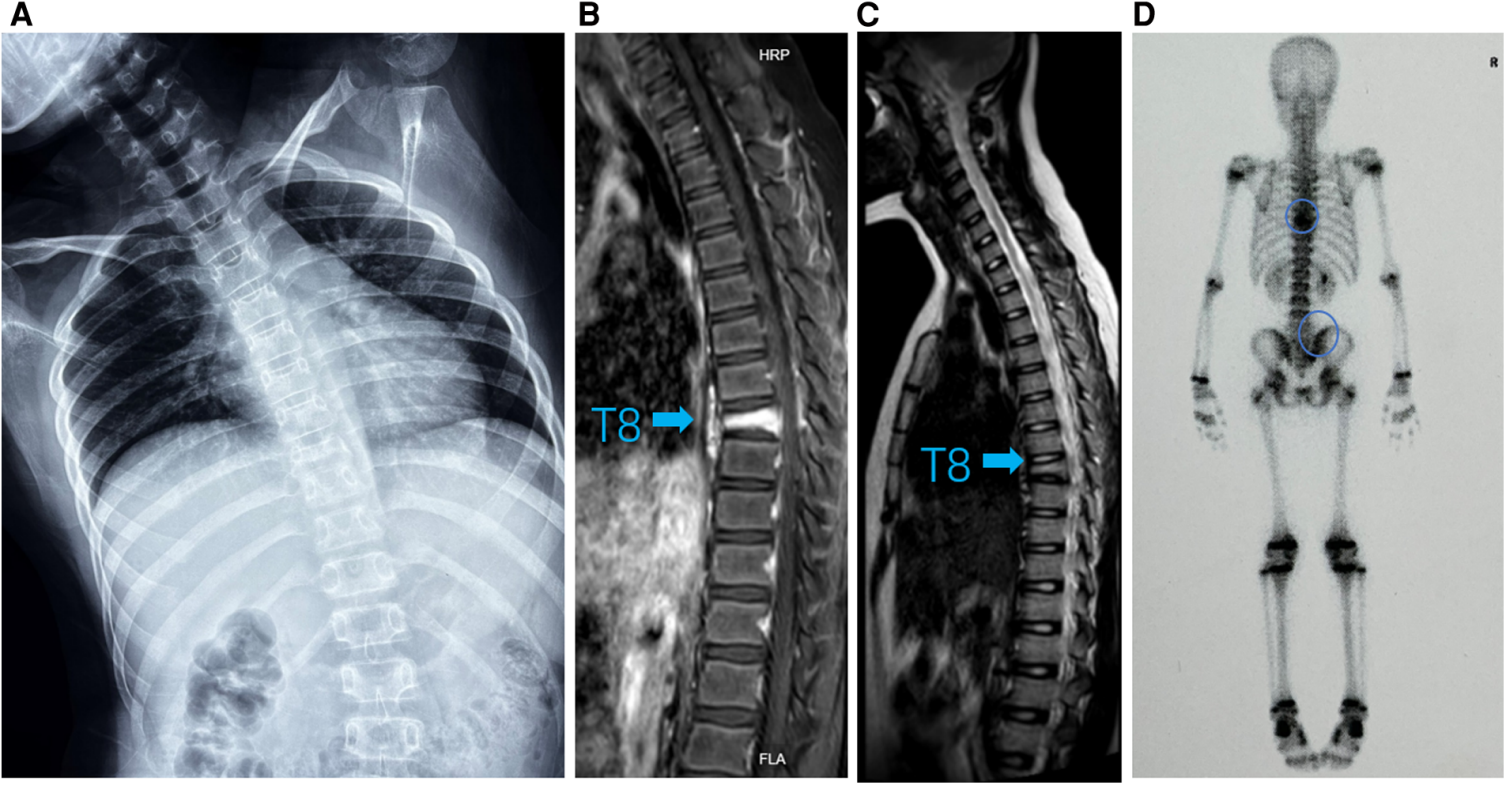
(A) Standing spinal x-rays showed heavier right scoliosis. (B) T1-weighted MRI image and (C) T2-weighted MRI image of the thoracic vertebra showed that the T8 vertebra had a compression fracture (arrow). (D) ^99m^Tc-MDP whole-body bone scintigraphy showing hot spots in T8/9 and right sacroiliac joint with posterior view (circle).
